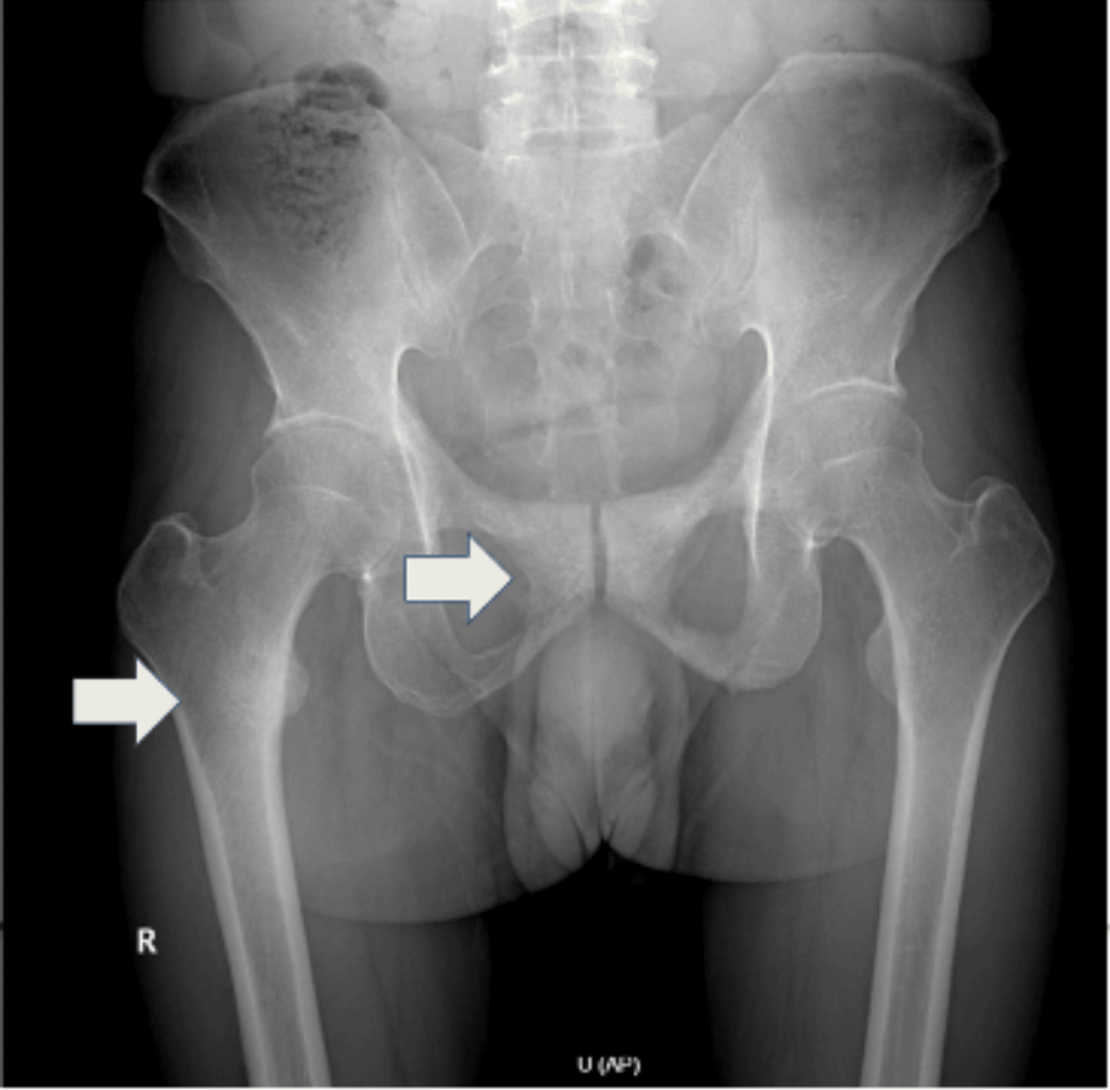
Plain radiograph of the pelvis and femur indicating metastatic lesions. White arrows show metastatic involvement of the right femoral lesser trochanter, bilateral ischium, and pubic rami.
